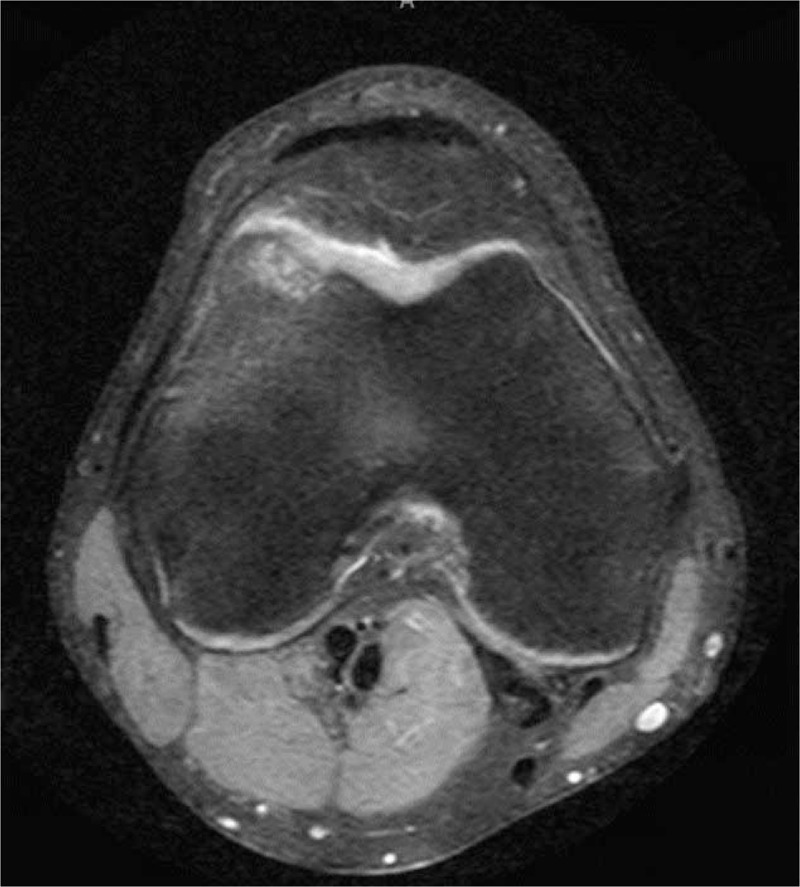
Axial magnetic resonance imaging demonstrating lateral trochlear osteochondritis dissecans lesion.
